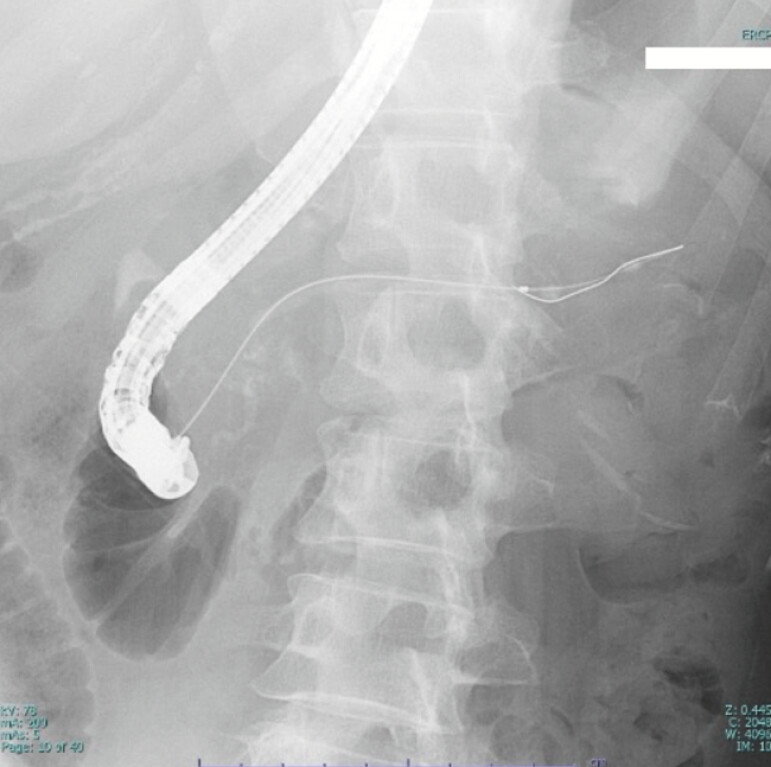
A radiograph showing the kinked guidewire in the pancreatic duct.
